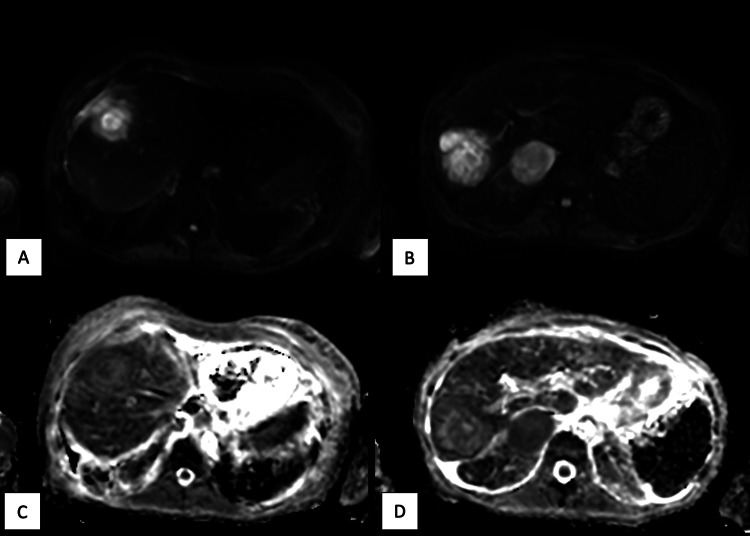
DWI MRI sequences of the superior (A) and inferior (B) lesions and ADC MRI sequences of the superior (C) and inferior (D) lesions showing internal hyperintensity on DWI with intermediate to low ADC values in the periphery of the lesions DWI: diffusion weighted imaging; ADC: apparent diffusion coefficient
